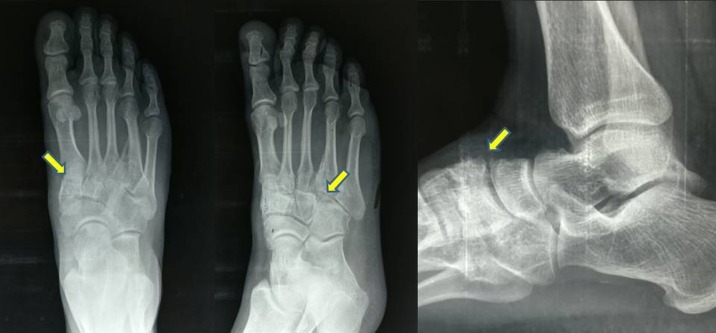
Pre-op radiograph (antero-posterior and lateral views) Shows arthritic first, second, and fourth TMT joints.
